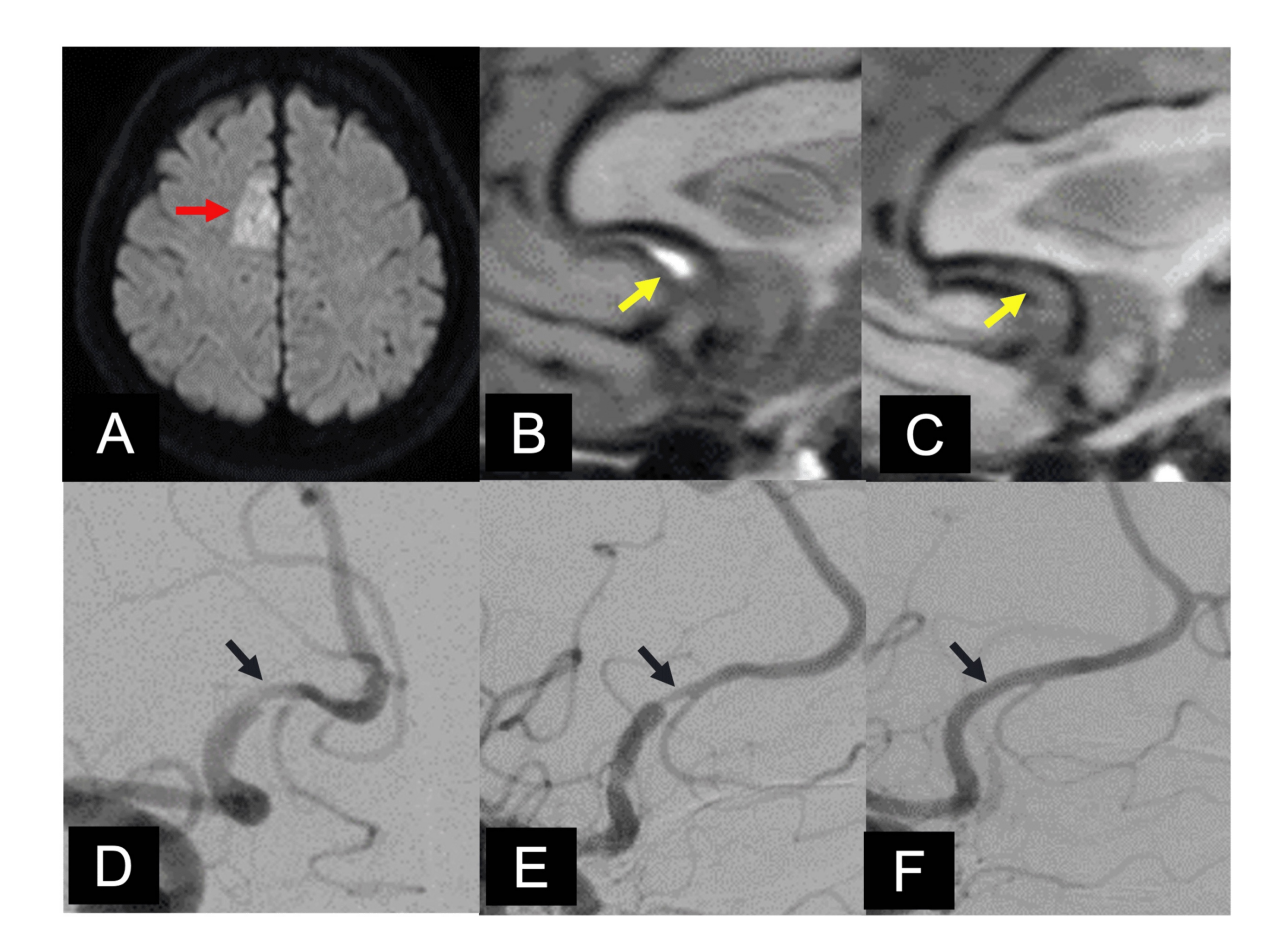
Case 8's radiological images A: The MRI showed acute cerebral infarction in the right anterior cerebral artery (ACA) territory (red arrow). B: We detected intramural hematoma consistent with the lesion using motion-sensitized driven-equilibrium sequence (MSDE). C: MSDE after three months showed the disappearance of intramural hematoma (yellow arrows). D: Digital subtraction angiography (DSA) at admission revealed irregular dilatation and stenosis in the right A2. E: DSA after two weeks showed changes in findings from distal to the bifurcation of the orbitofrontal artery to proximal to the bifurcation of the frontopolar artery. F: DSA after three months showed normalization of ACD findings (blue arrows). A2: Extends from the anterior communicating artery (AComA) to the region of the rostrum and genu of the corpus callosum
